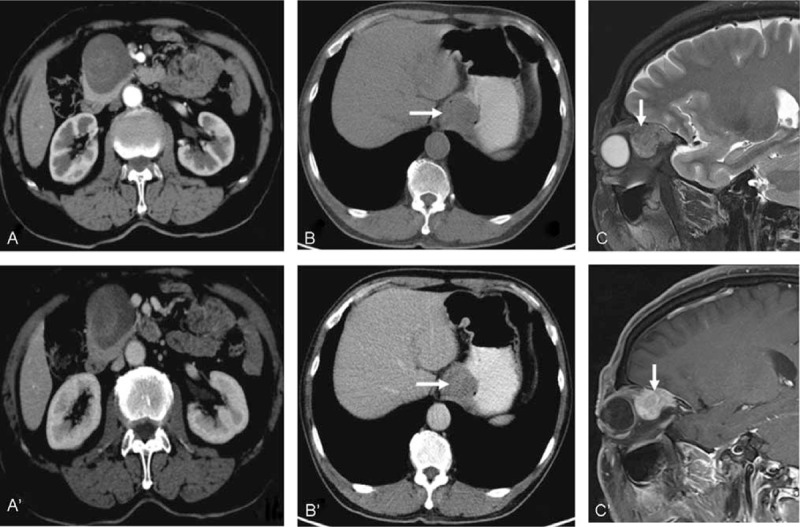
Typical features of rare locations of SFTs. A, A’: A 71-year-old man with a pancreatic SFT. Contrast-enhanced CT showed an oval, well-defined heterogeneous mass (arrows) with marked heterogeneous enhancement (A, A’). B, B’: A 69-year-old man with an esophageal SFT. CT images before (B) and after (B’) contrast-enhancement showed an oval, well-defined homogeneous mass with marked homogeneous enhancement (arrow). C, C’: A 57-year-old male patient with an orbital SFT. T1-weighted MRI scans before (C) and after (C’) contrast-enhancement showed an oval, well-defined heterogeneous mass (arrow) with marked heterogeneous enhancement. CT = computed tomography, MRI = magnetic resonance imaging, SFTs = solitary fibrous tumors.
